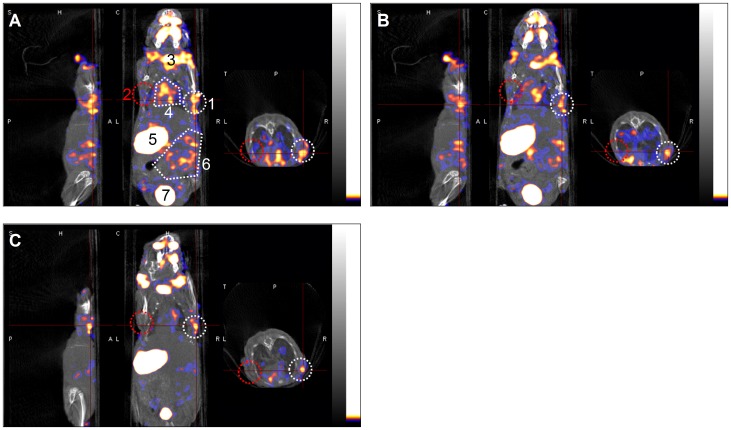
In vivo* imaging of Bac-NIS-infected hUCB-MSCs transplantation with NanoSPECT/CT. A: Overlapping SPECT and CT images at 30 min after administration of 300 µCi (11.1 MBq) Na^125^I. The right axilla (white circular area) was transplanted with Bac-NIS-infected hUCB-MSCs (MOI = 200, 1×10^7^ cells) and showed a high radioiodide uptake, while the left axilla (red circular area) was transplanted with mock-infected cells as a control and showed no obvious radioiodide uptake. From left to right side are, respectively, the coronal, sagittal and horizontal sections. All CT images are shown with a grey palette, and all SPECT images are shown with a warm palette. B and C: SPECT/CT images at 60 min and 120 min after radioiodide administration. Abbreviations: CT, computed tomography; SPECT, single photon emission computed tomography; 1, Bac-NIS-infected hUCB-MSCs; 2, mock-infected hUCB-MSCs; 3, thyroid; 4, heart; 5, stomach; 6, intestinal area; 7, bladder.
